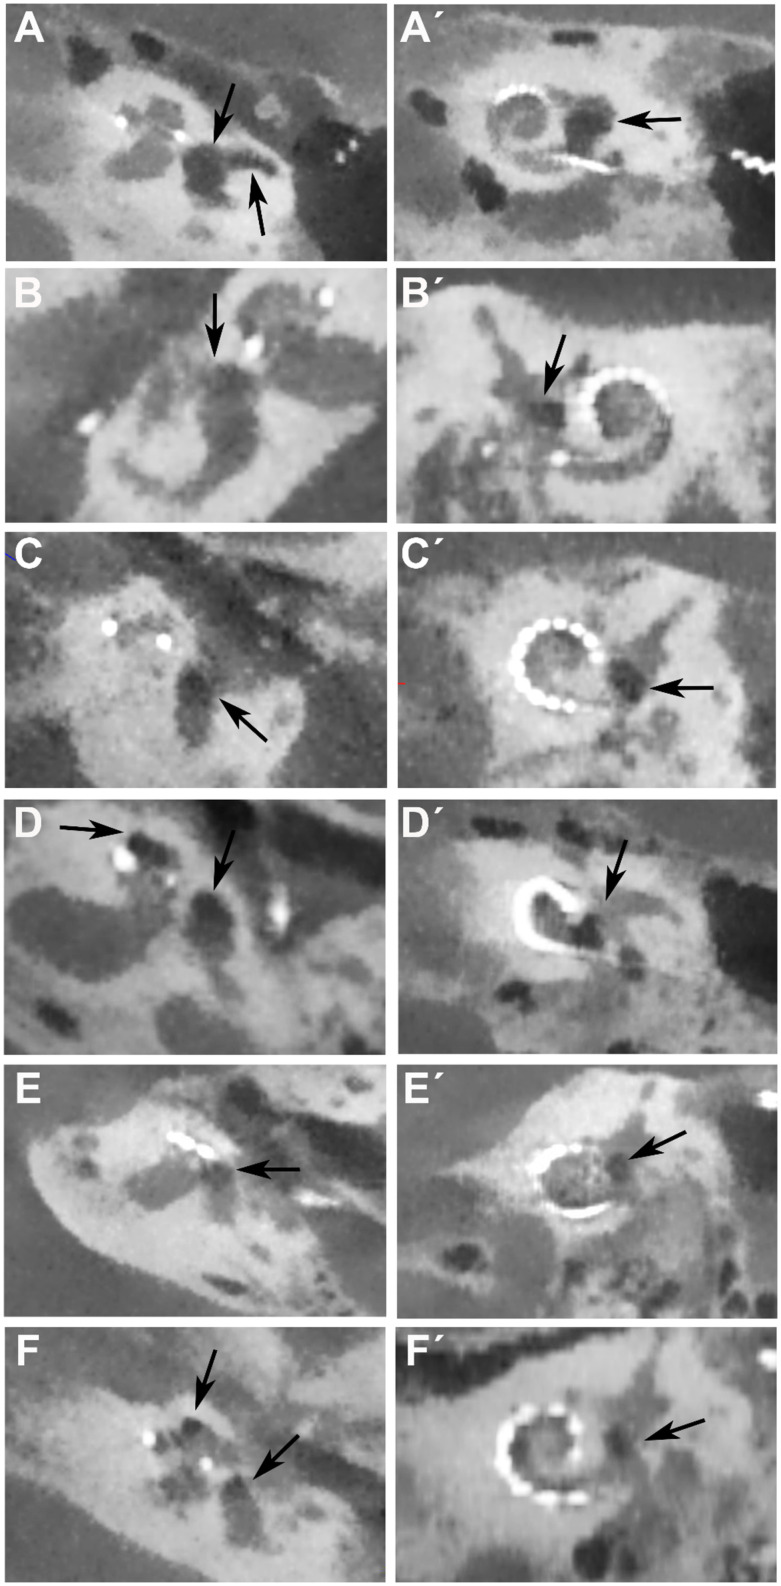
Postoperative CBCT images for electrode position control in cochlea view of the six patients (A–F) with vertigo and on air suspected changes in the vestibulum after CI surgery. Arrow shows localization of air in the vestibule in the axial view (A–F) and coronal view (A´-F´). In patient (A) there is additional air in the lateral semicircular canal and in patients (D,F) additional air is found in the apical turn of the cochlea (second arrows).
